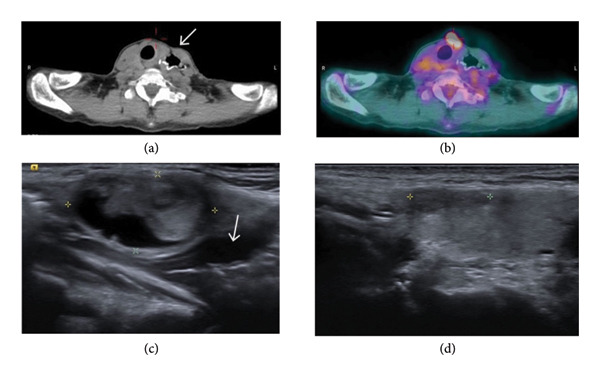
(a, b) PET/CT revealed a high SUVmax value of the left thyroid nodule with misregistration artifact and retained metallic clips at the reconstructed esophagus with colon interposition at the same anatomic level. Sonography of the same patient with follicular neoplasm before RFA (c) and 6 months after RFA (d) (arrow: reconstructed esophagus).
